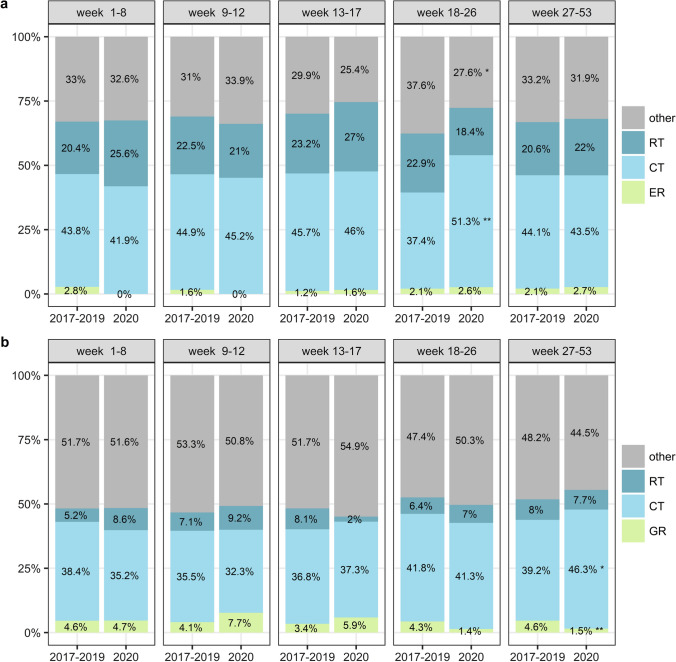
Plot of the overview of treatments given in the palliative setting stratified by period of diagnosis where a shows the treatments of patients with incurable esophageal cancer and b shows the treatments in patients with incurable gastric cancer. ER; esophageal resection with or without other treatments, GR; gastric resection with or without other treatments, CT; chemotherapy without resection and with or without other treatments, RT; radiotherapy without resection or chemotherapy
